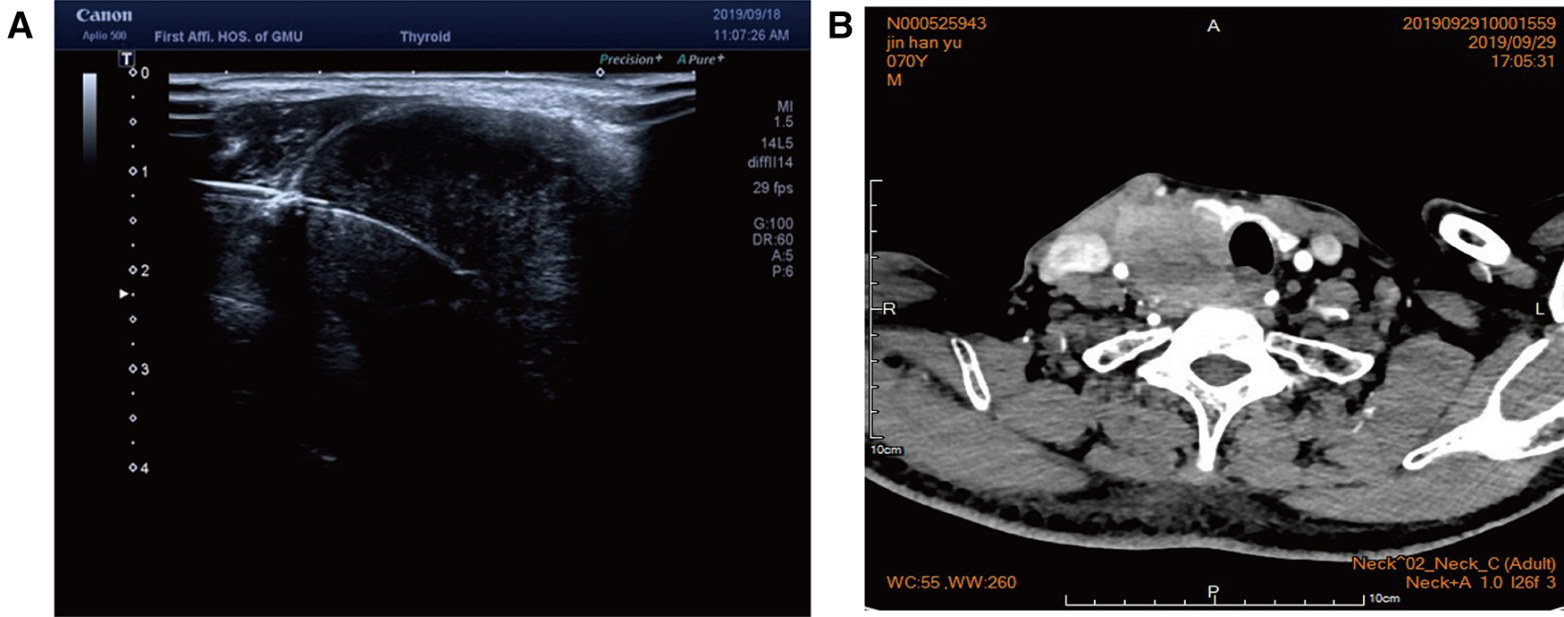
(A) Color ultrasound showed hypoecho in the right lobe of the thyroid gland, about 44 mm × 40 mm × 49 mm in size, with unclear boundary. (B) Contrast-enhanced CT of the neck showed a mass in the right lobe of the thyroid, with infiltration of the surrounding internal jugular vein, common carotid artery, trachea, and esophagus.
